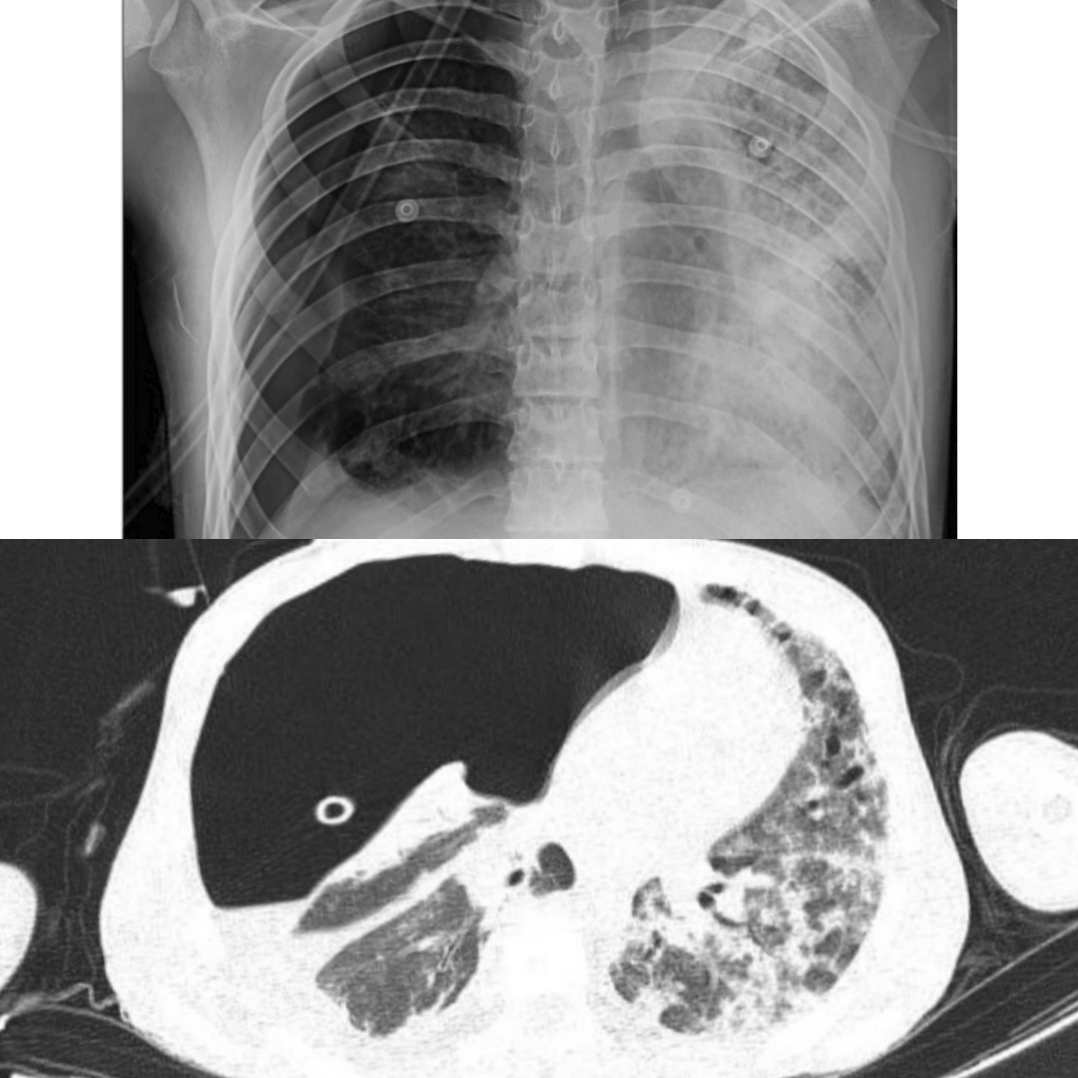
Chest X‐ray and Chest CT scan showing pneumothorax on the right lung with mediastinum shifted to the left.
